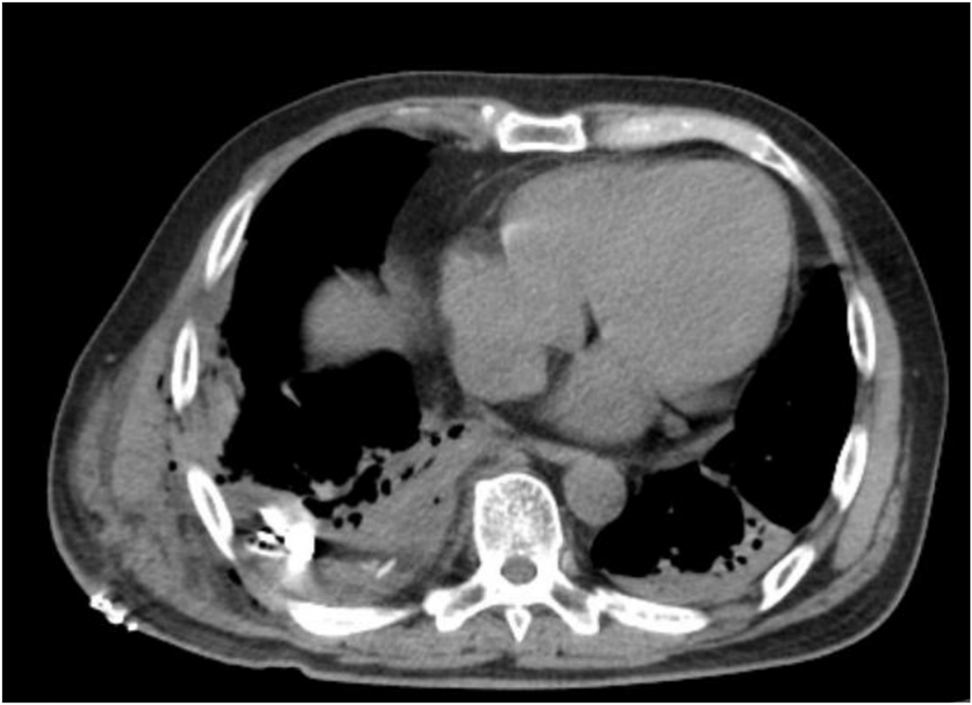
Chest CT findings: Most of the hematoma has been removed. Artifacts from TachoSil Tissue Sealing sheet (CSL Behring) and Polyglycolic acid sheet were visible
